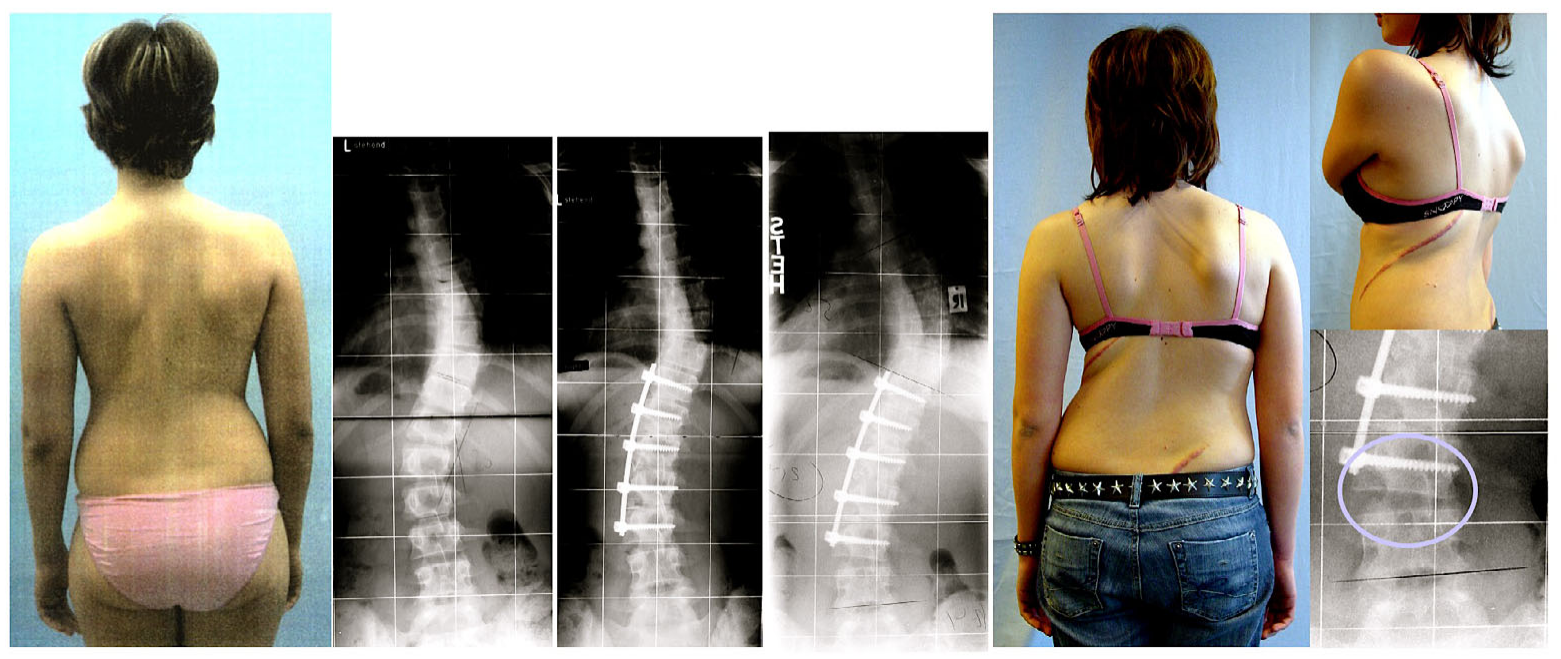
Clinical deterioration after surgery for Adolescent Idiopathic Scoliosis (AIS). Radiography and clinical appearance of a 15-year old female AIS patient with a double curve of initially 32 degrees thoracic and 28 degrees lumbar. The lumbar curve has been fused although this leads to decompensation and clinical deterioration. The risk for curve progression in this relatively mature patient (15 years of age, three years postmenarchial) was minimal before undergoing surgery!
